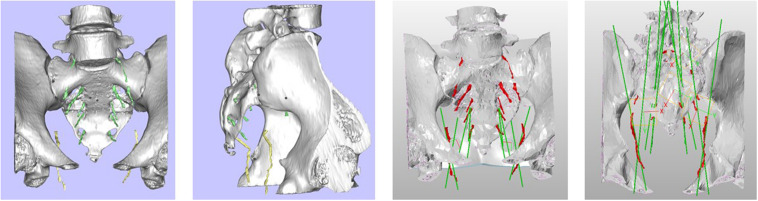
3D printing guide plate CT and MRI data fusion modeling.
